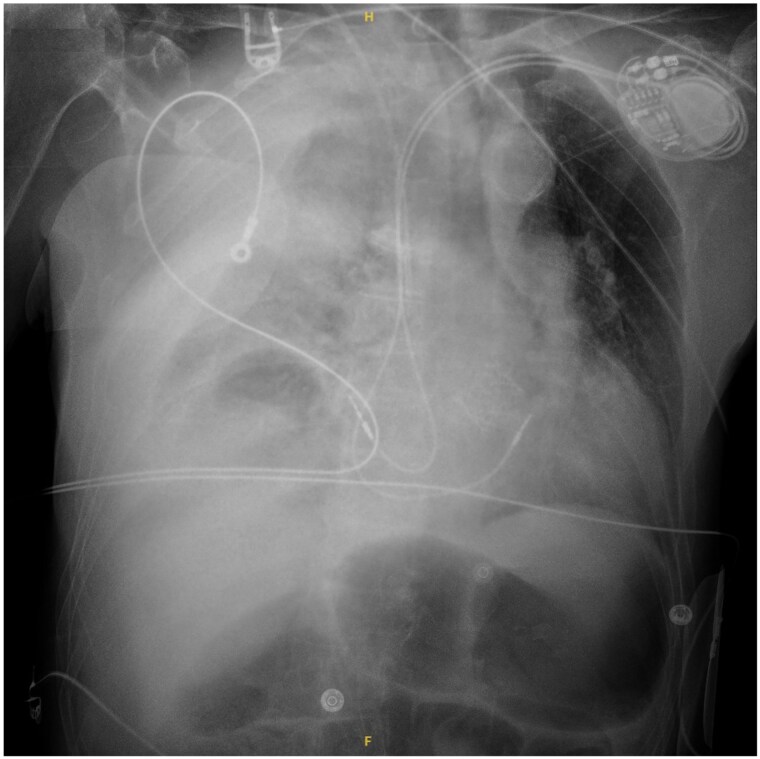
Chest radiograph obtained 12 h after pacemaker implantation showing significant worsening of the effusion with homogeneous opacification of the right hemithorax.
